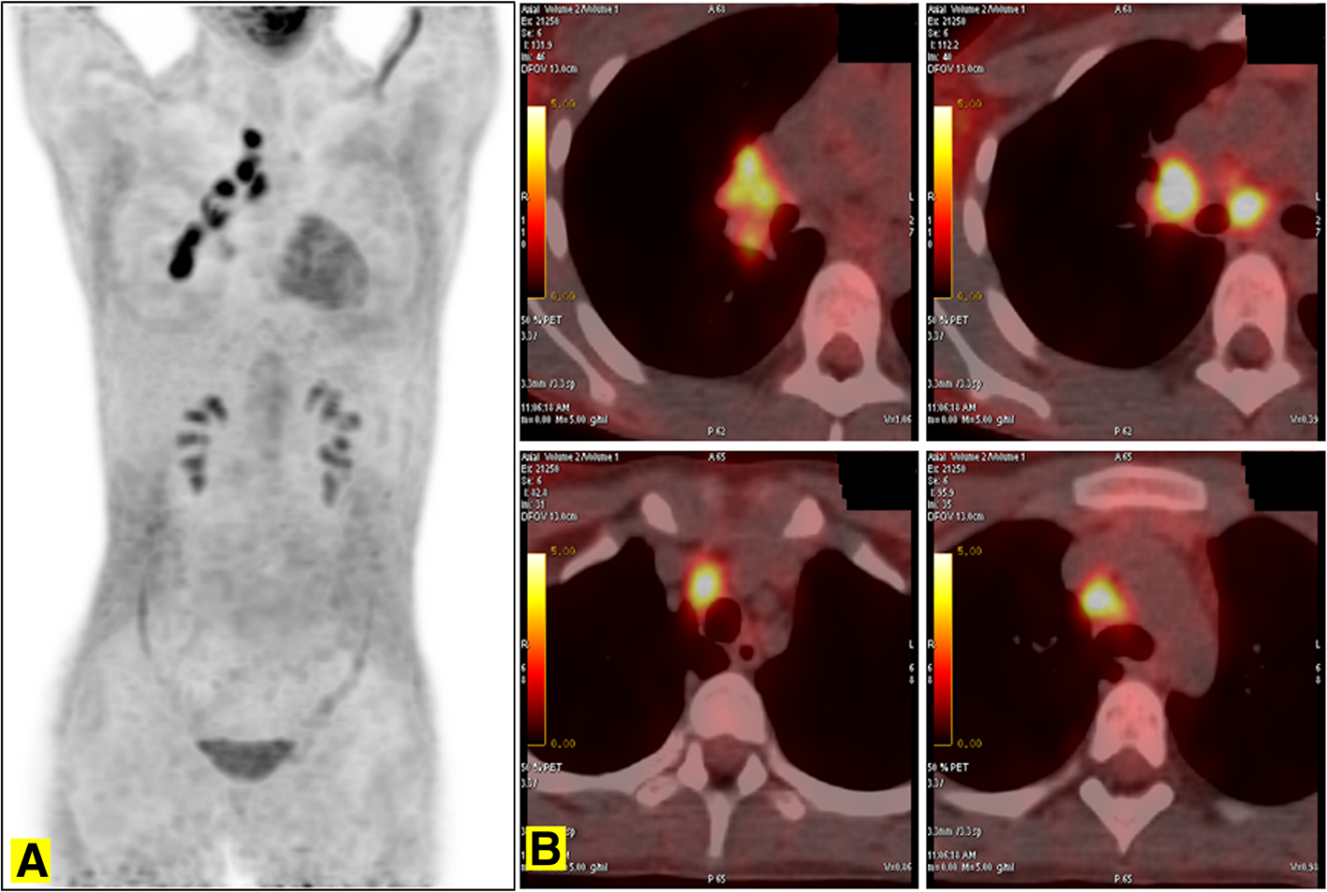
Thoracic positron emission tomography scan (a) and positron emission tomography/computed tomography scans (b) performed for the staging of lung adenocarcinoma
